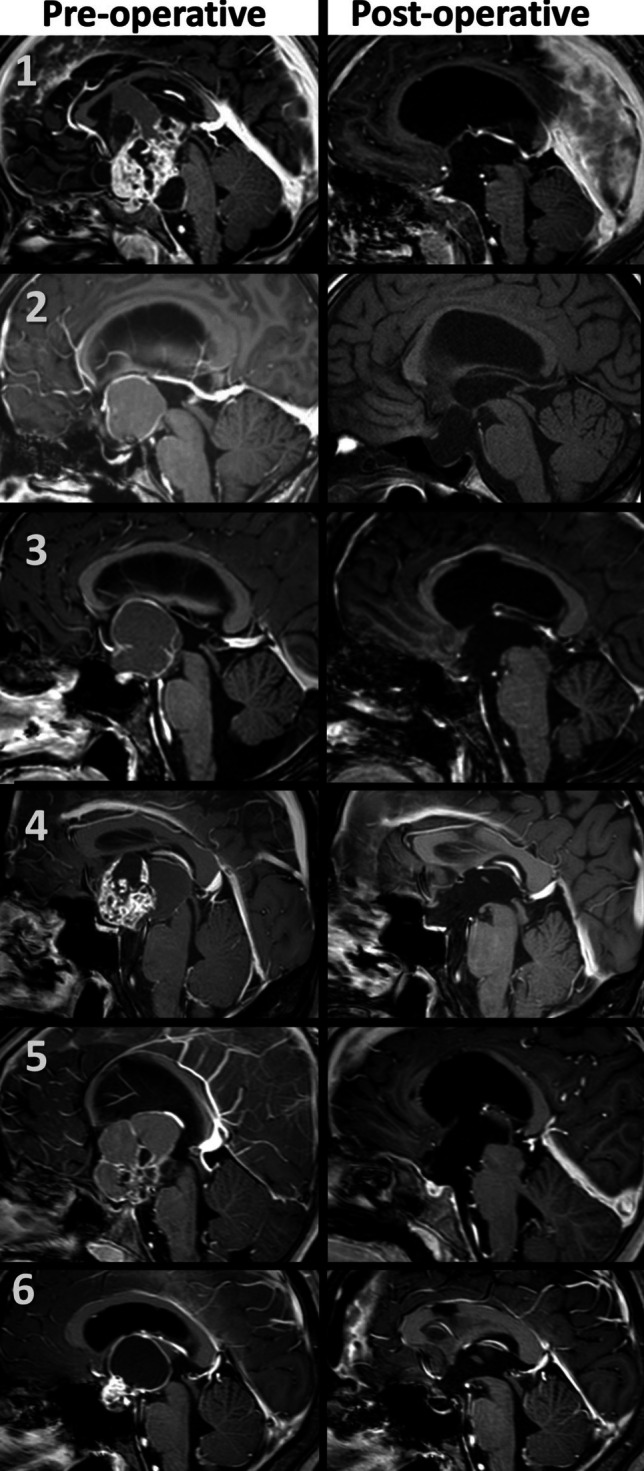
Preoperative and postoperative sagittal contrast-enhanced MRI images of the first 6 patients
